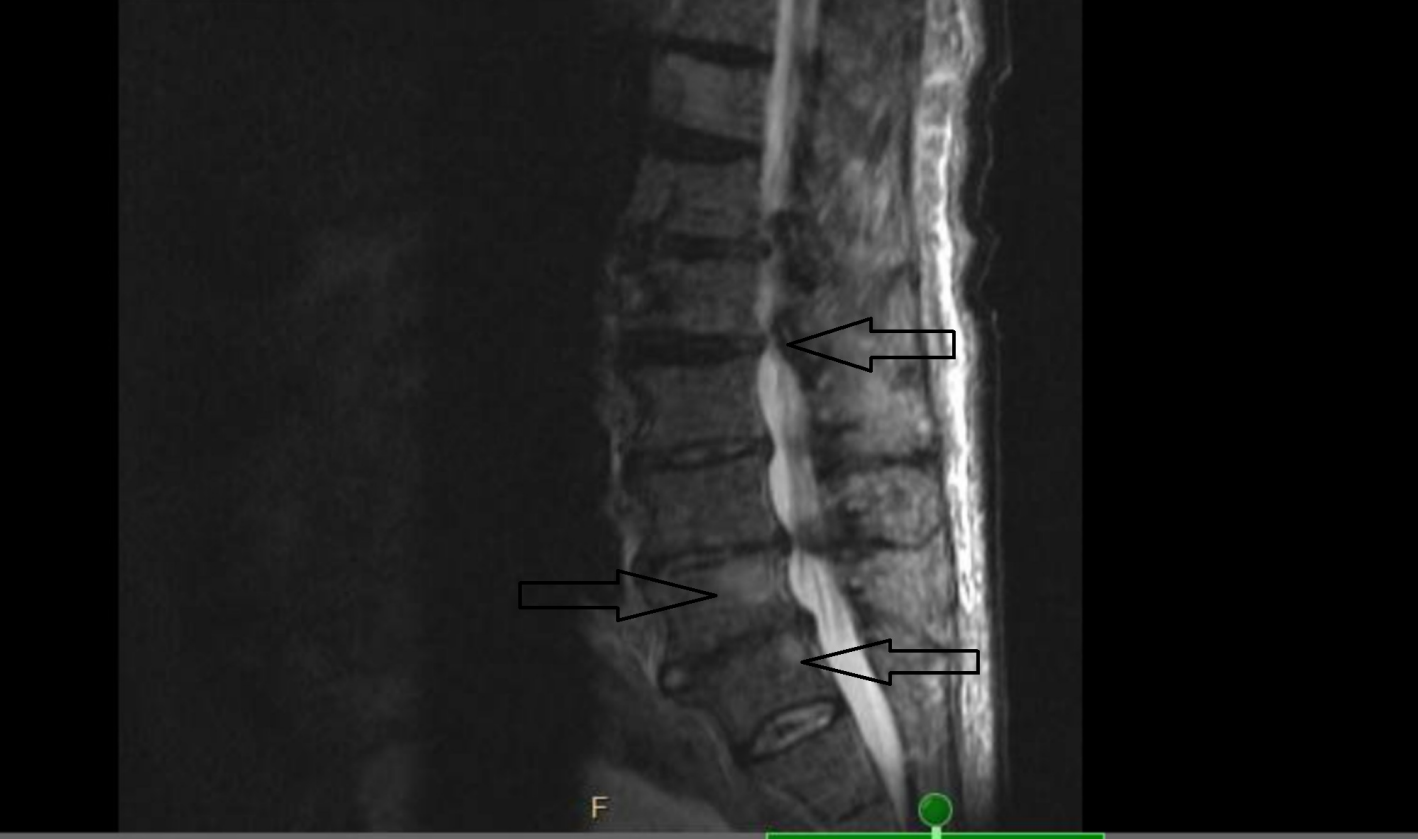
Magnetic resonance imaging (MRI) of the lumbar spine Showing indeterminate cord compression (marked by an arrow) and multiple bone lesions, two of them marked by arrows.
